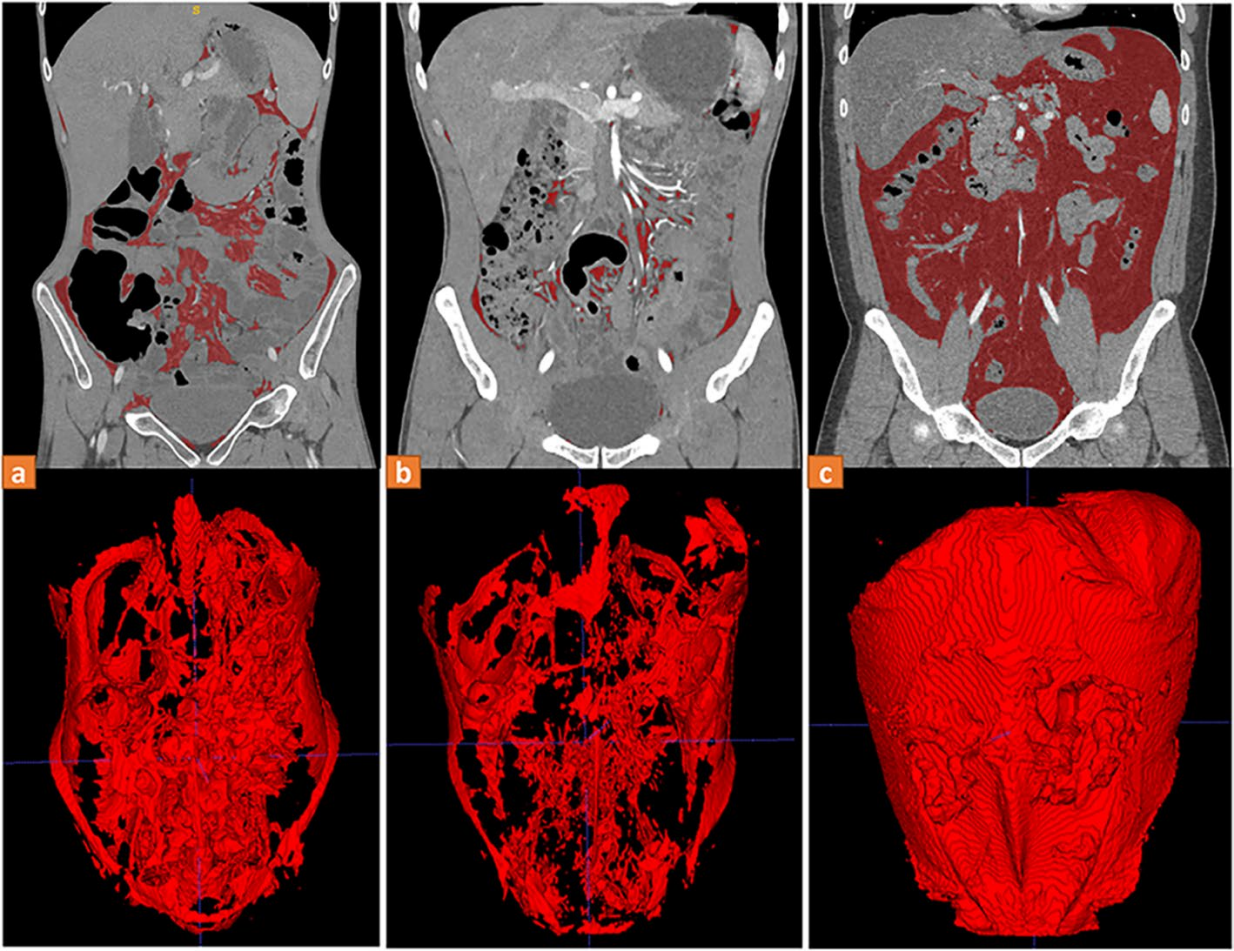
Coronal CT images of three patients diagnosed with CD, UC, and acute appendicitis, and their visceral adipose tissue was marked red. a A 24-year-old male CD patient with a BMI of 12.35 kg/m^2^ presenting with ileocolonic CD. The average VFA within the lumbar region was 22.46 cm^2^ with a CV of 66.39%. b A 30-year-old male UC patient with a BMI of 17.82kg/m^2^, presenting with mild UC. The average VFA was 58.84 cm^2^, and the CV was 30.15%. c A 32-year-old male appendicitis patient had a BMI of 27.71kg/m^2^. The average VFA was 161.30 cm^2^, and the CV was 28.86%. CD, Crohn’s disease; UC, ulcerative colitis; BMI, body mass index; VFA, visceral fat area; CV, coefficient of variation
